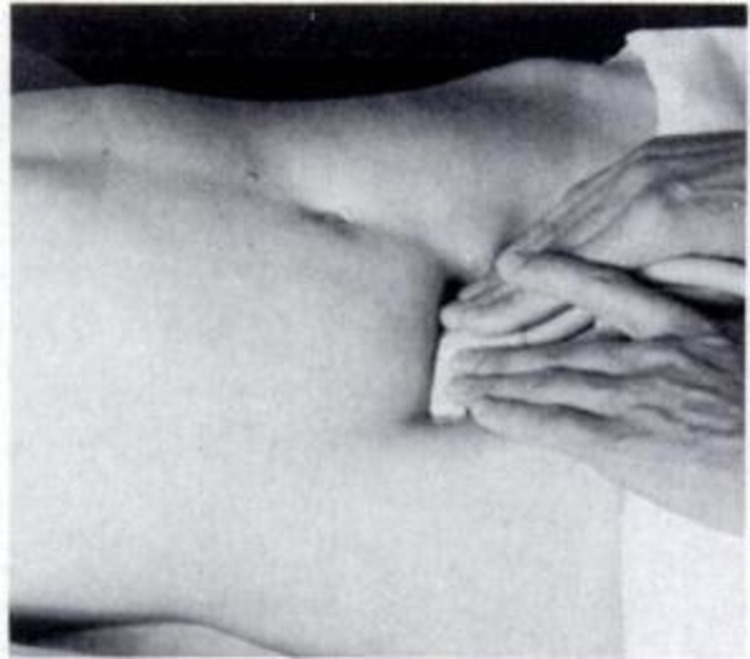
Ultrasound graded compression technique by Puylaert. Puylaert JB. Acute appendicitis: US evaluation using graded compression. Radiology. 1986 Feb;158(2):355-60.
